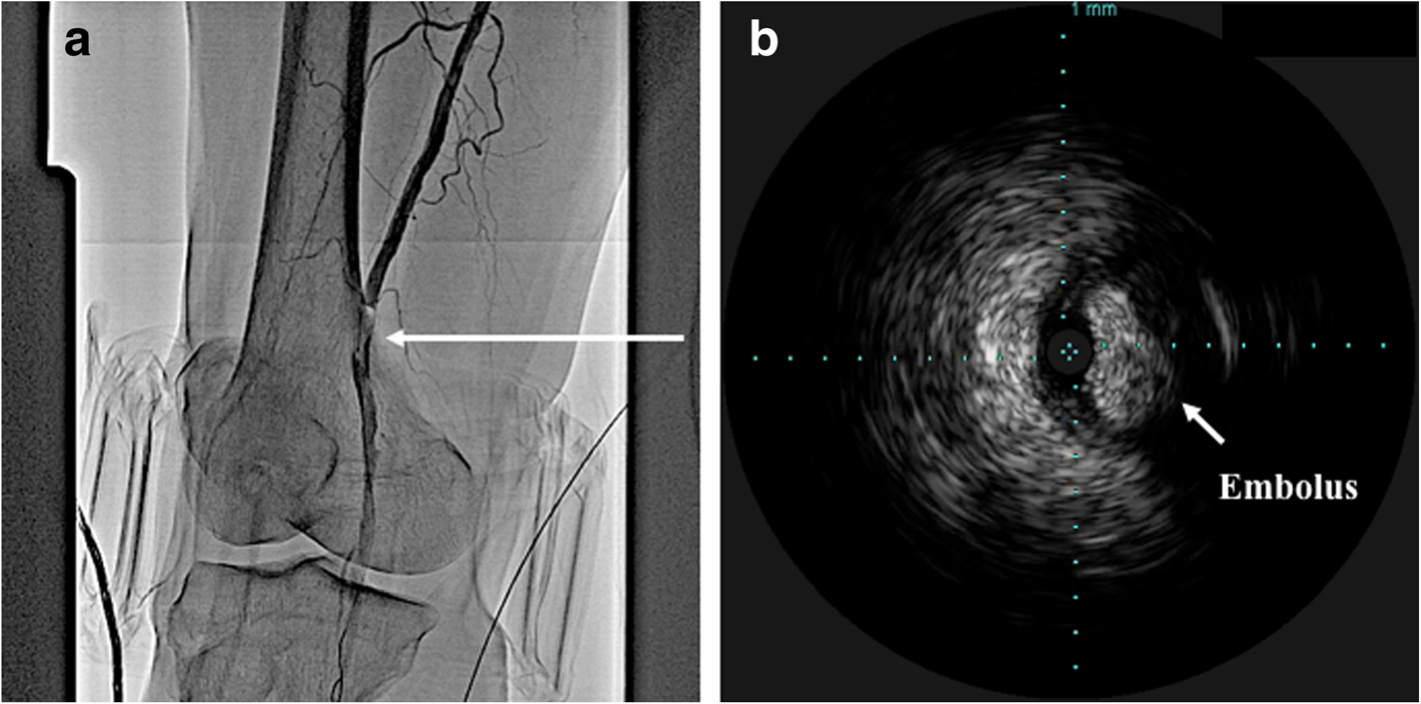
a The angiography showed subtotal occlusion (arrow) at the proximal popliteal artery. b Intravascular ultrasound image demonstrated a smooth-surfaced high-density homogenous structure (arrow) which was suspected as a polyglycolic acid fiber plug material of the EXOSEAL vascular closure device
